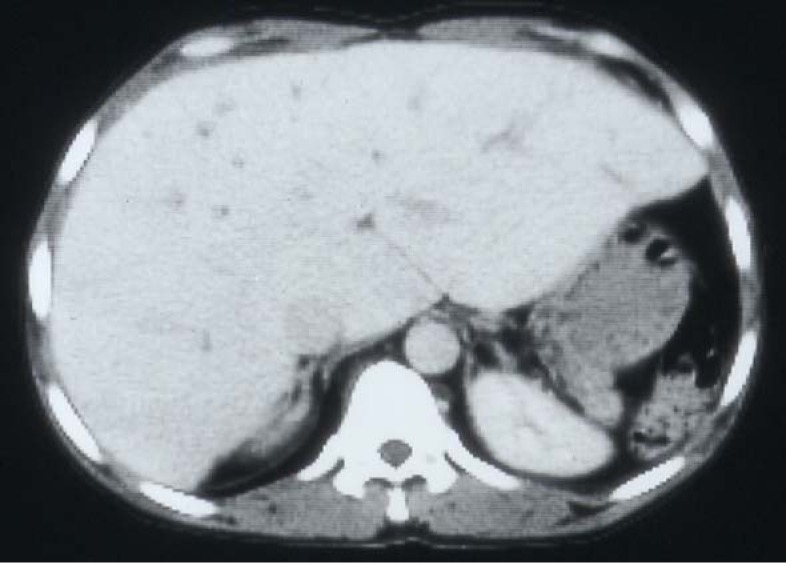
CT-scan of the abdomen showing the liver with iron overload in a patient with SCA and multiple blood transfusions.
